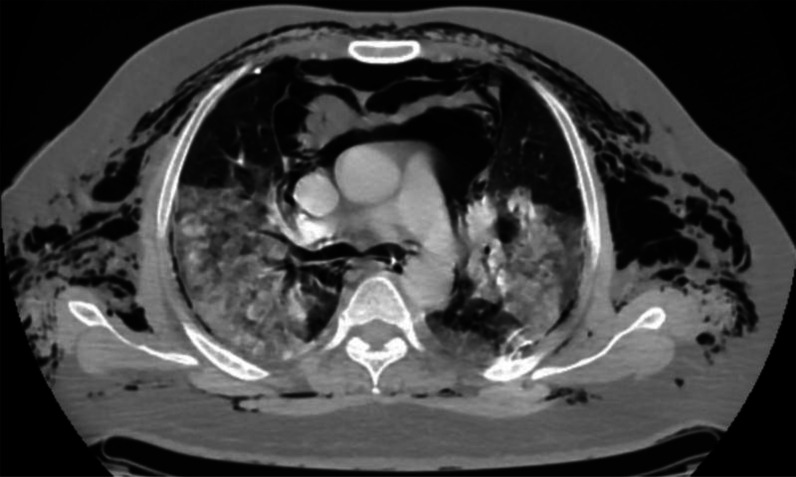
Axial CT image with HRCT window showing extensive subcutaneous emphysema in the chest wall on both sides and extensive air foci seen in the mediastinum.
